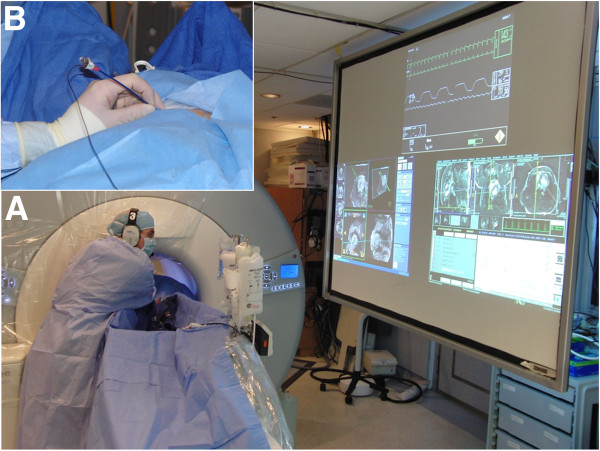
The interventional CMR environment used here. Panel (A) shows the operator leaning into the 70 cm bore to establish transthoracic access during real-time CMR. LCD projectors display instantaneous hemodynamics, scanner control, and CMR. Acoustic noise suppression headsets allow communication with staff in the control room. Inset (B) shows the access port with the table moved out of the scanner, viewed from the left leg.
